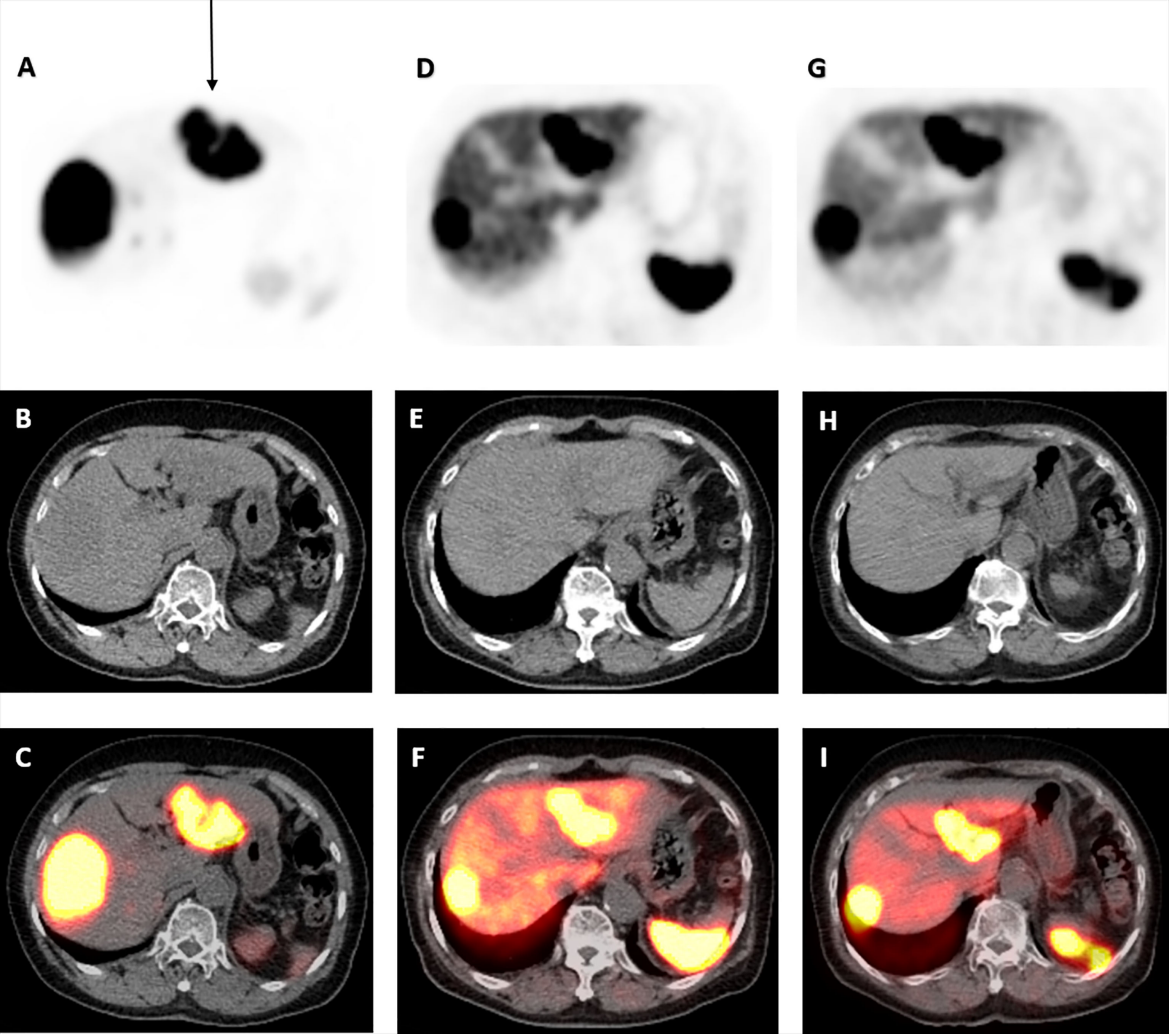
68Ga-DOTATATE PET/CT scan images of dominant left liver lobe lesion pre- and post-177 Lu-DOTATATE. Axial view images of ^68^Ga-DOTATATE PET/CT scan demonstrating interval reduction in size in dominant left liver lobe lesion (arrow) on PET, low-dose CT, and PET/CT fusion (top to bottom) from baseline, to 1 and 4 years after ^177^Lu-DOTATATE therapy (left to right). Physiological uptake in the spleen and remainder of the liver is also visualized.
